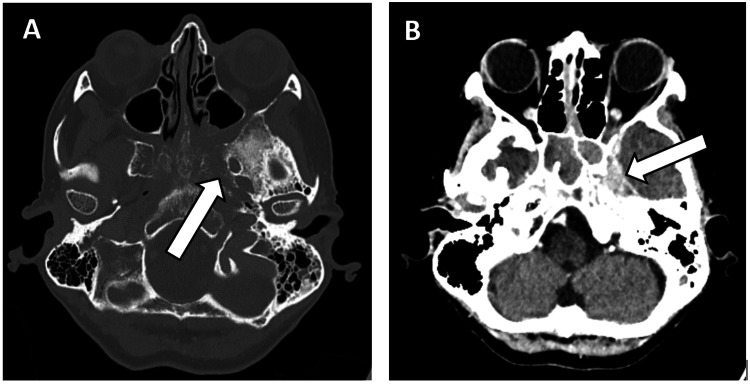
A head CT scan demonstrating a dense material within the left sphenoid sinus, with erosion of the wall of the left sphenoid sinus and adjacent sphenoid bone (A). Additionally, there is a high-density collection/enhancing soft tissue in the left Meckel's cave (B), adjacent to the eroded left sphenoid bone.
